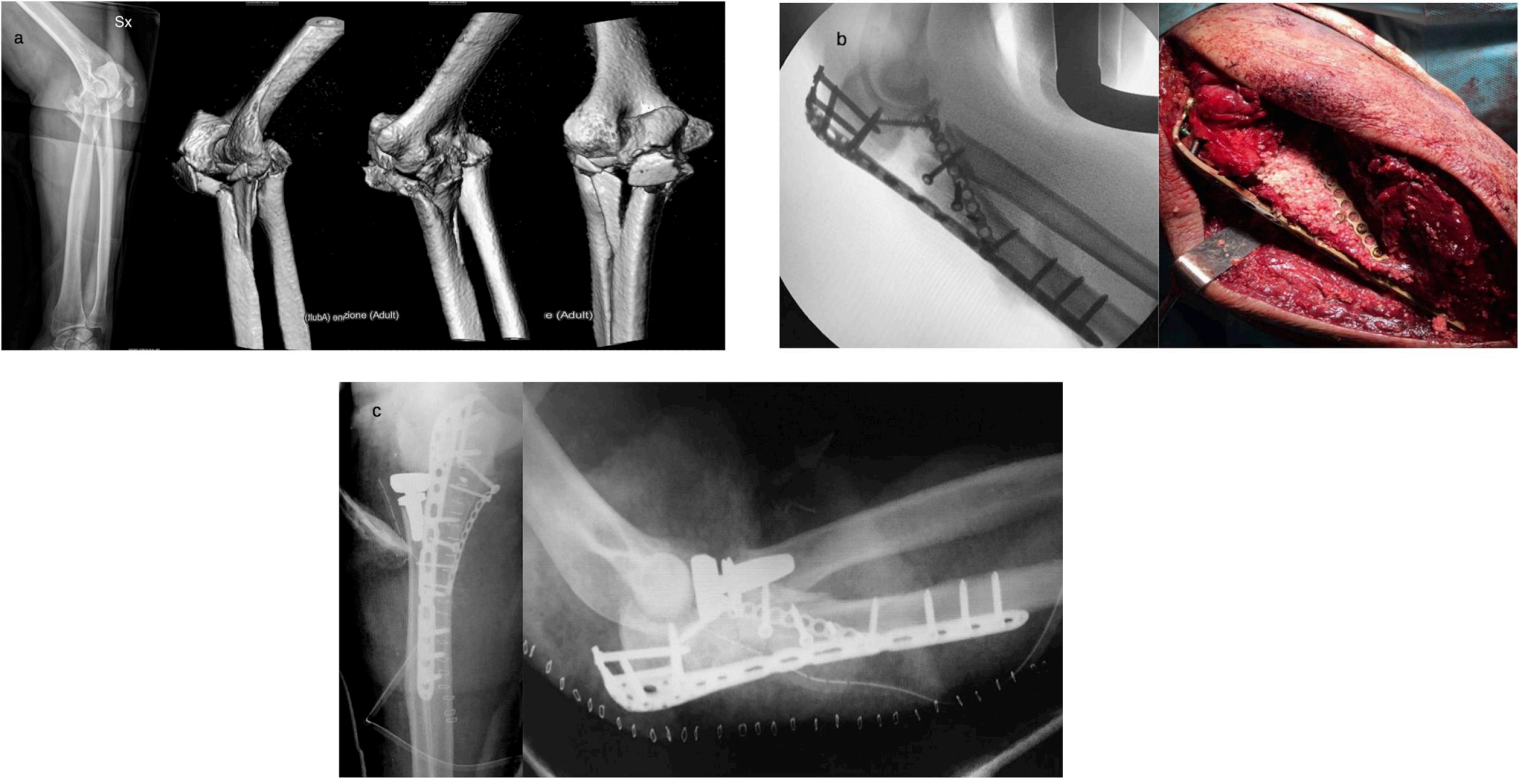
a) 3D-CT scan: high comminution of proximal ulna, sigmoid notch; large fragment of coronoid process. Comminuted fracture of the radial head. The patient was a 58-year-old woman. b) Intraoperative fluoroscopy and surgical field: The Taylor-Sham approach and standard Kocher approach - filling of cancellous gap with autograft obtained from the excision of radial head fragments and morselized homologous bone. c) Post-operative radiograph: Pre-contoured locking compression plate, free screws, headless 18-mm screw and 2.0 buttress plate for coronoid fracture; Radial head prosthesis.
